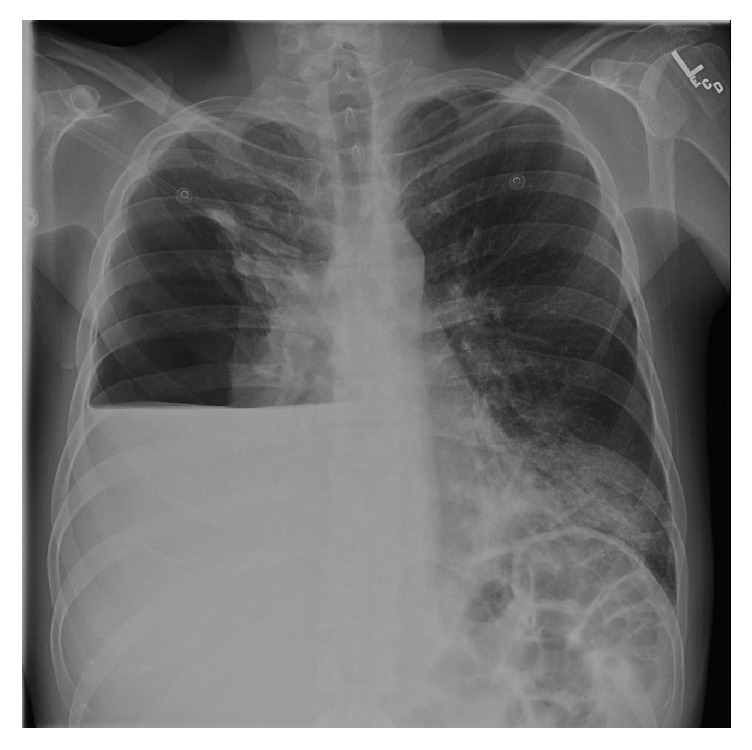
Chest X-ray after thoracentesis showing large right pneumothorax with collapse of the lung into the hilar region. Large right hemithorax air-fluid level. Increased airspace consolidation within the left lower lobe.
